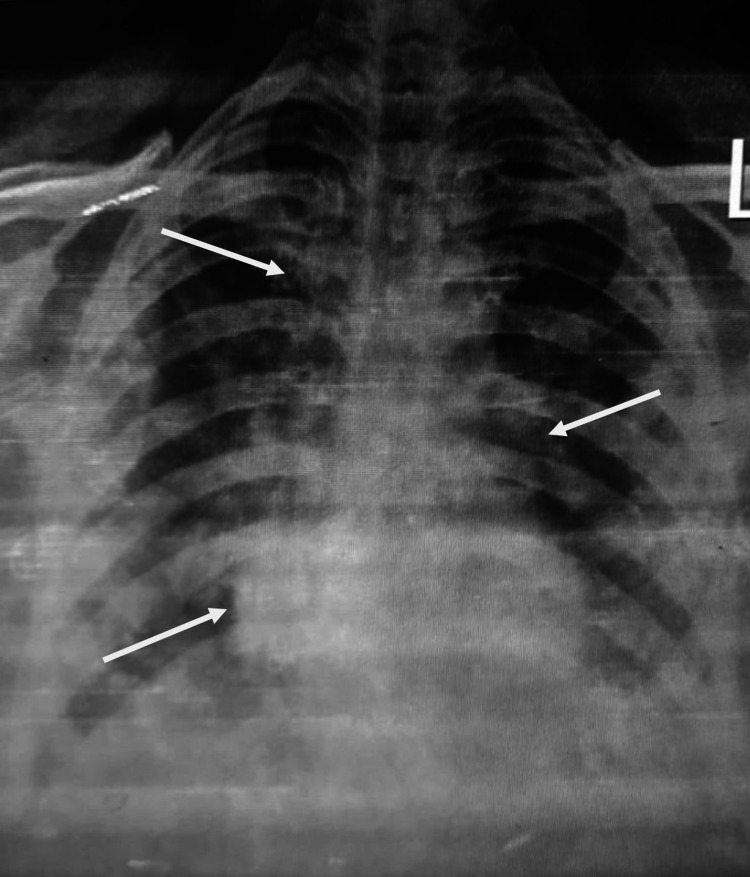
Chest X-ray showing infiltrates and pneumonic patches in both lungs.
